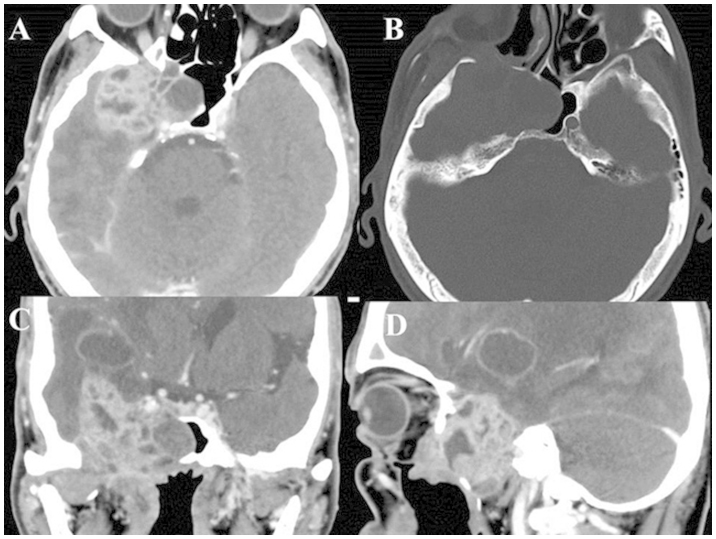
CT scan obtained on admission. (A) Axial, (C) coronal and (D) sagittal enhanced CT scans showing a mass occupying the maxillary sinus extending to the temporal base. (B) Bone imaging showing a right middle cranial base deficiency. CT, computed tomography.
